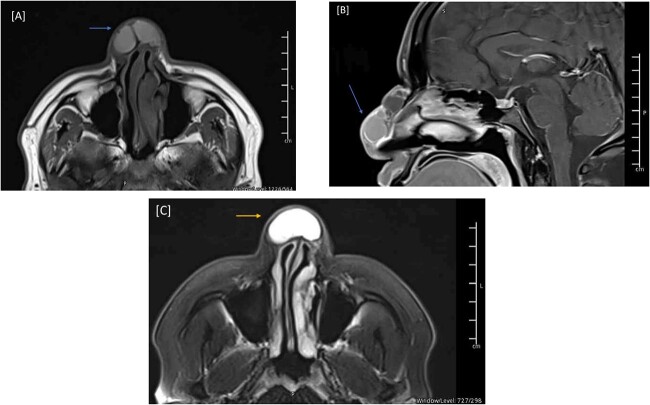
MRI of the head, and paranasal sinuses (Pre-Op). (A) There is a lobulated multilocular cystic lesion seen at the dorsum of the nose, some of the cysts are showing bright signal intensity in T1 (arrow) and T2/FLAIR (C) (arrow) representing high proteinaceous content. No connection to the cranial cavity, features are likely presenting dermoid cyst. (B) The lesion shows peripheral postcontrast enhancement. No diffusion restriction.
